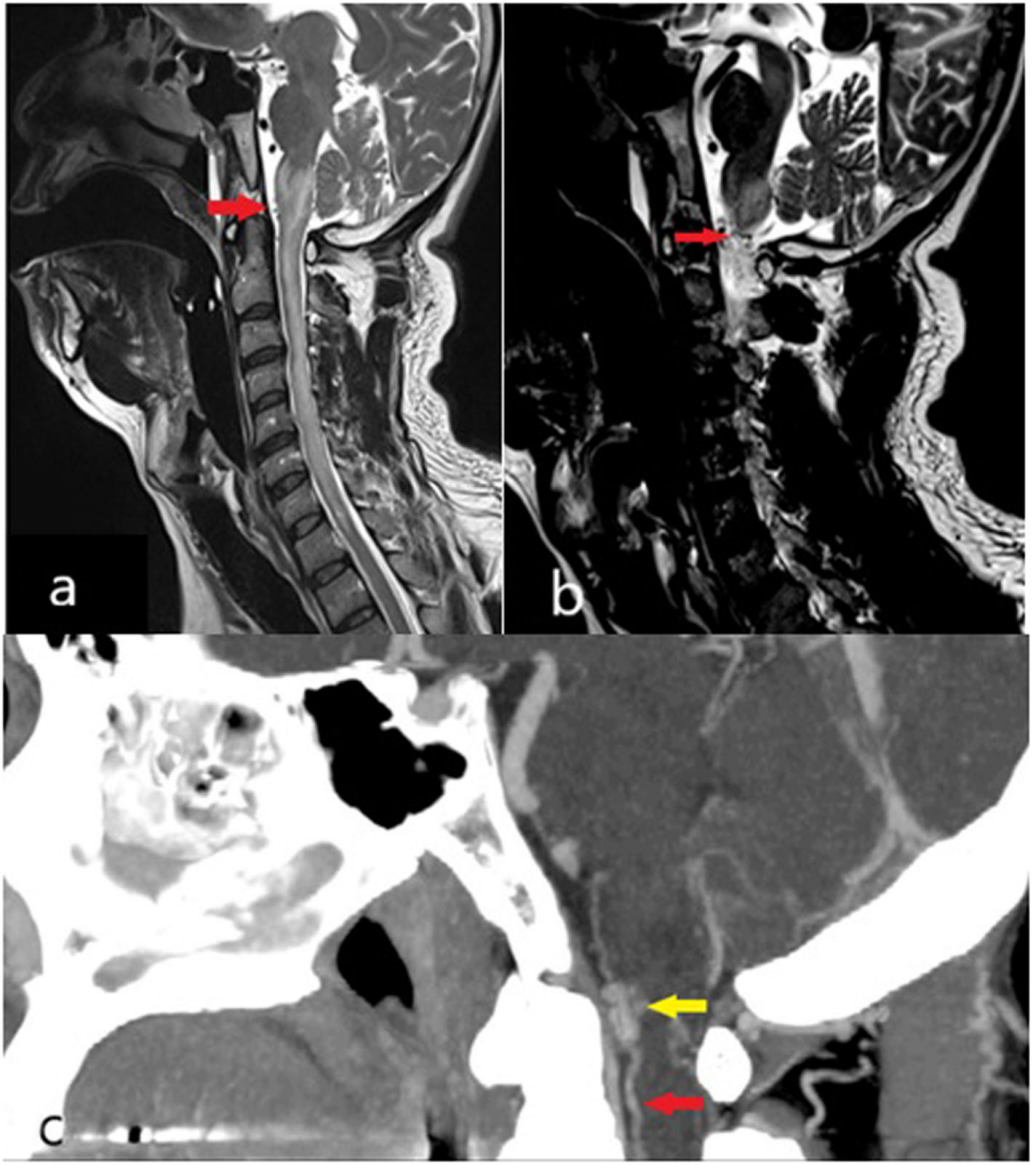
a and b MRI of patient 1 demonstrated longitudinal extensive T2 hyperintensity from medulla to C7 spinal cord level with dilated perimedullary veins anterior to the cord at the cervical region (red arrow). c. CT angiogram demonstrated arterio-venous fistula (yellow arrow) at C1 level and the anterior dilated perimedullary veins (red arrow)
